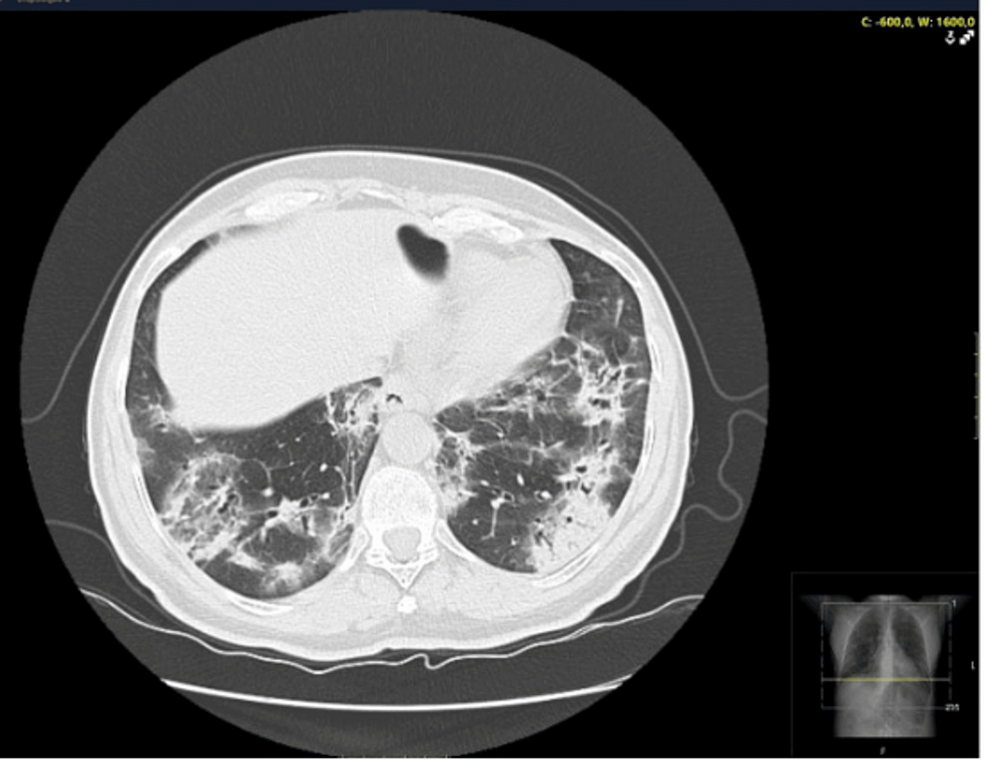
First chest CT scan at the time of the presentation in the Emergency Department.
